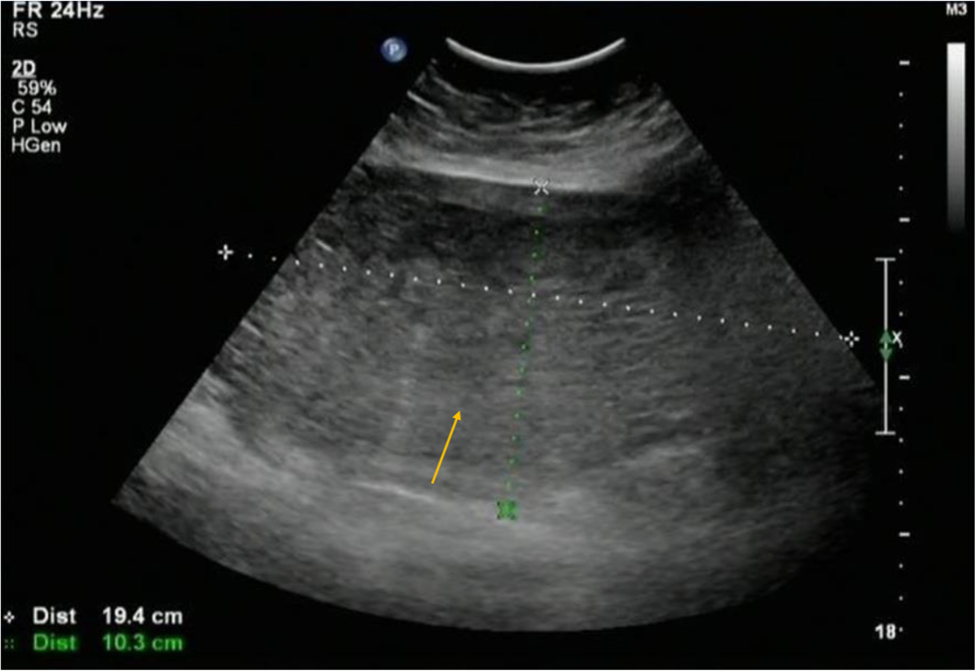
Ultrasound images showing a huge molar mass and faveolate subechoic area (arrow) in the uterine cavity.
